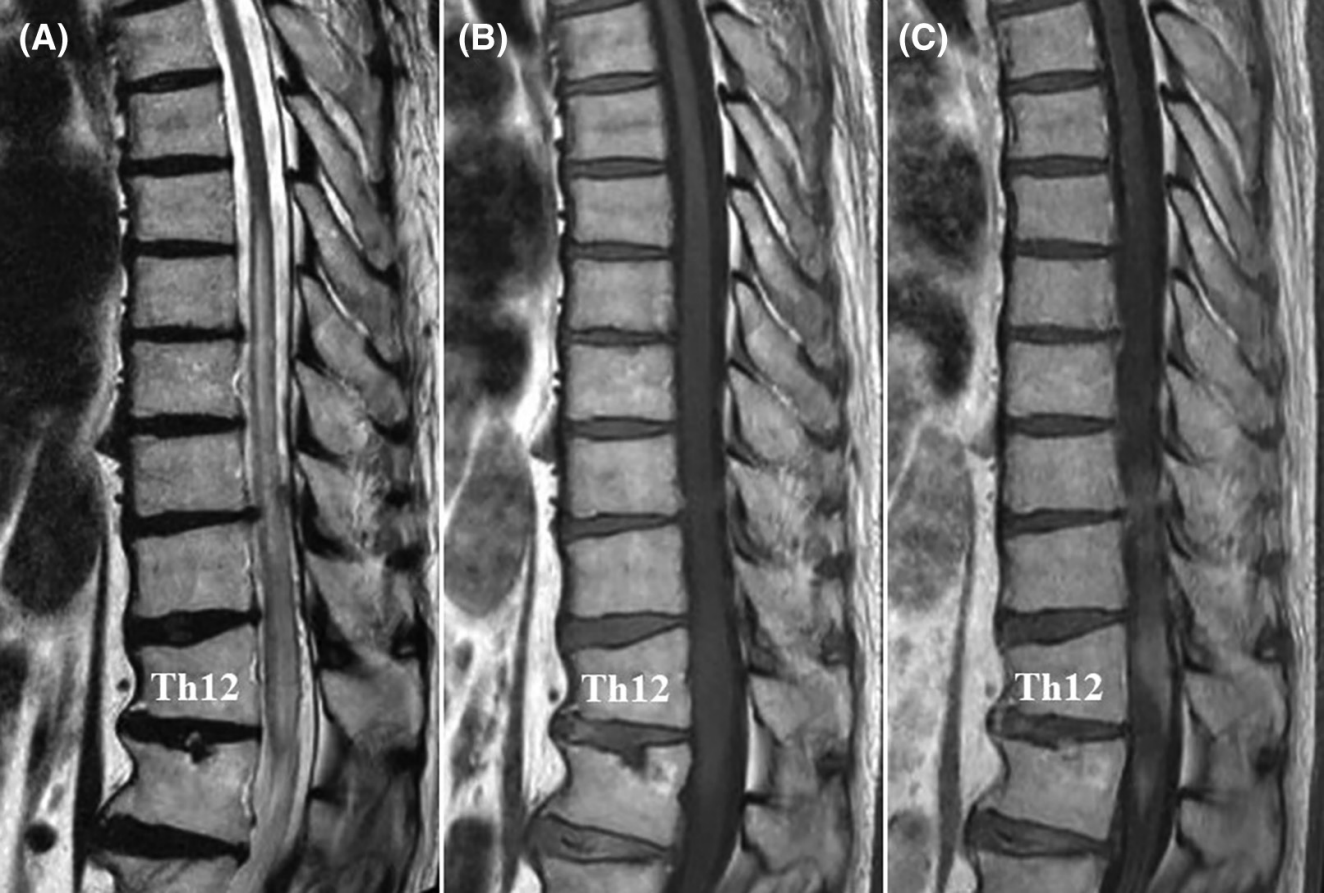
Preoperative thoracic magnetic resonance imaging (MRI). (A) Sagittal T2‐weighted MRI showing spinal cord edema and flow voids around the cord at the Th7–L1 level. (B) Sagittal T1‐weighted MRI showing spinal cord edema. (C) Sagittal contrast‐enhanced MRI showing heterogeneous enhancement of the spinal cord at the Th10–L1 level.
